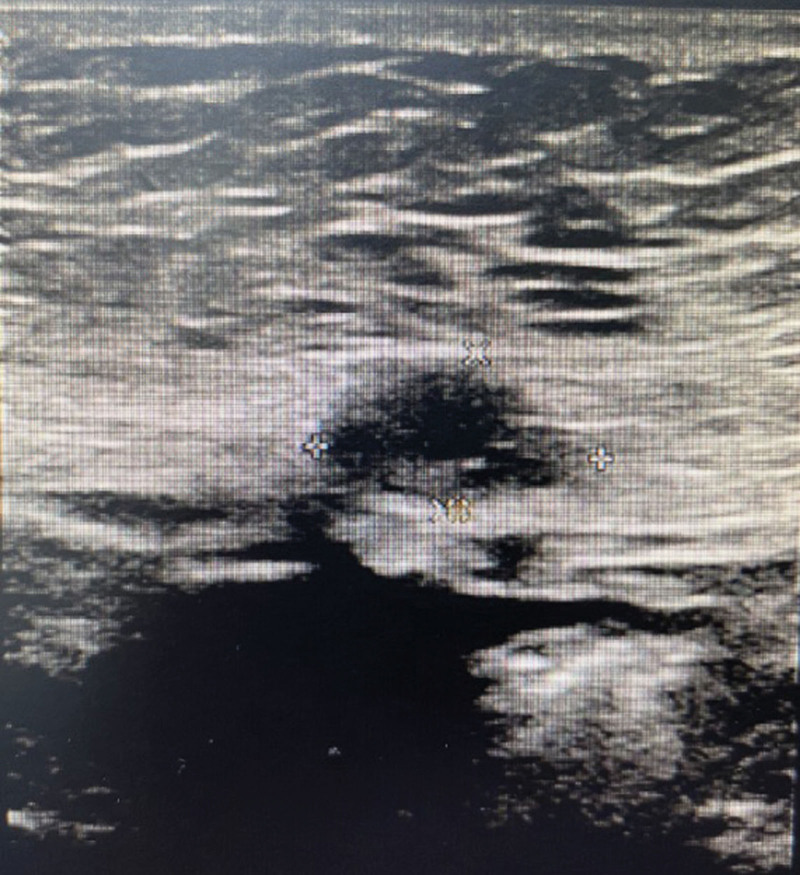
Ultrasound of abdominal wall masses showed a hypoechoic mass within the muscle layer of the lower left abdomen, measuring approximately 22 mm × 4 mm × 10 mm, located 16.6 mm from the body surface.
